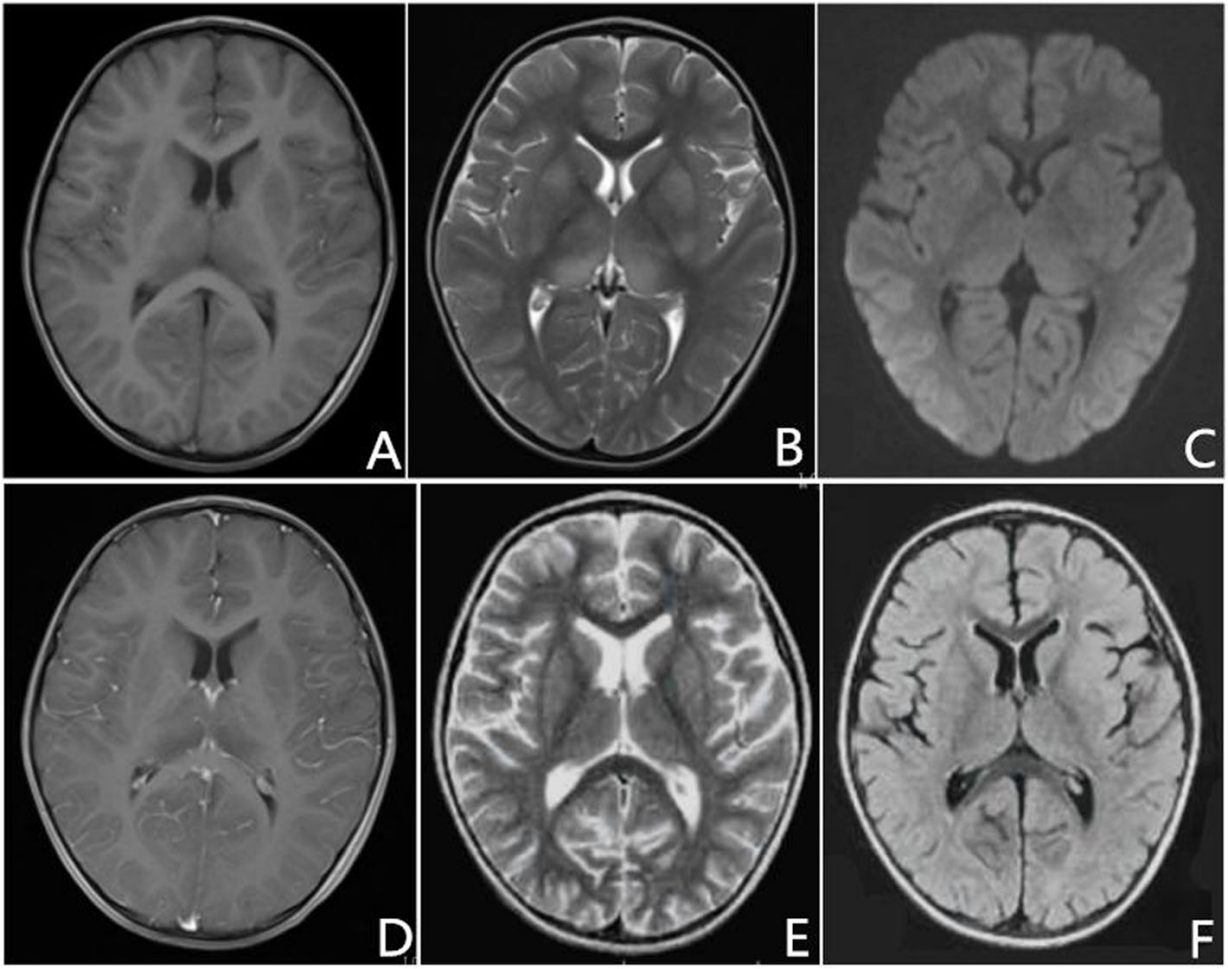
a, b On day 2 after admission, brain MRI showed bilateral lenticular nucleus and thalamus lesions, hypointense on T1-weighted image and hyperintense on T2-weighted image. c None of the lesions showed diffusion restriction. d Post contrast T1 axial image showed bilateral leptomeningeal enhancement. e, f On day 17 after admission, a follow-up MRI examination demonstrated no residual lesions on T2-weighted and FLAIR sequences
